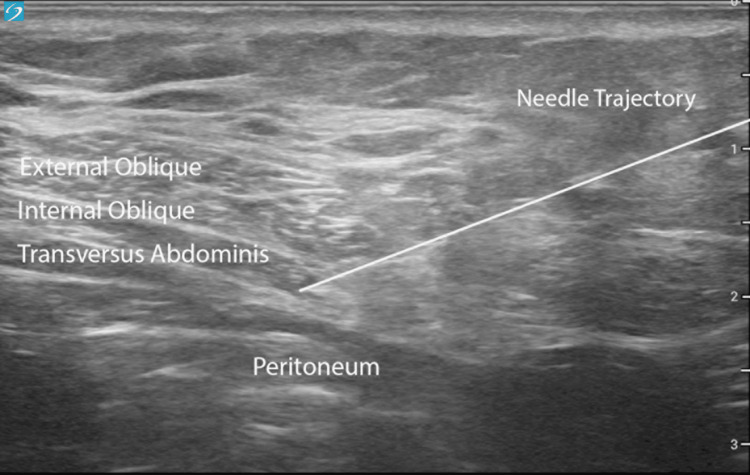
In-plane ultrasound visualization of the needle approach to the TAP during PNS lead placement TAP, transverse abdominis plane; PNS, peripheral nerve stimulation
